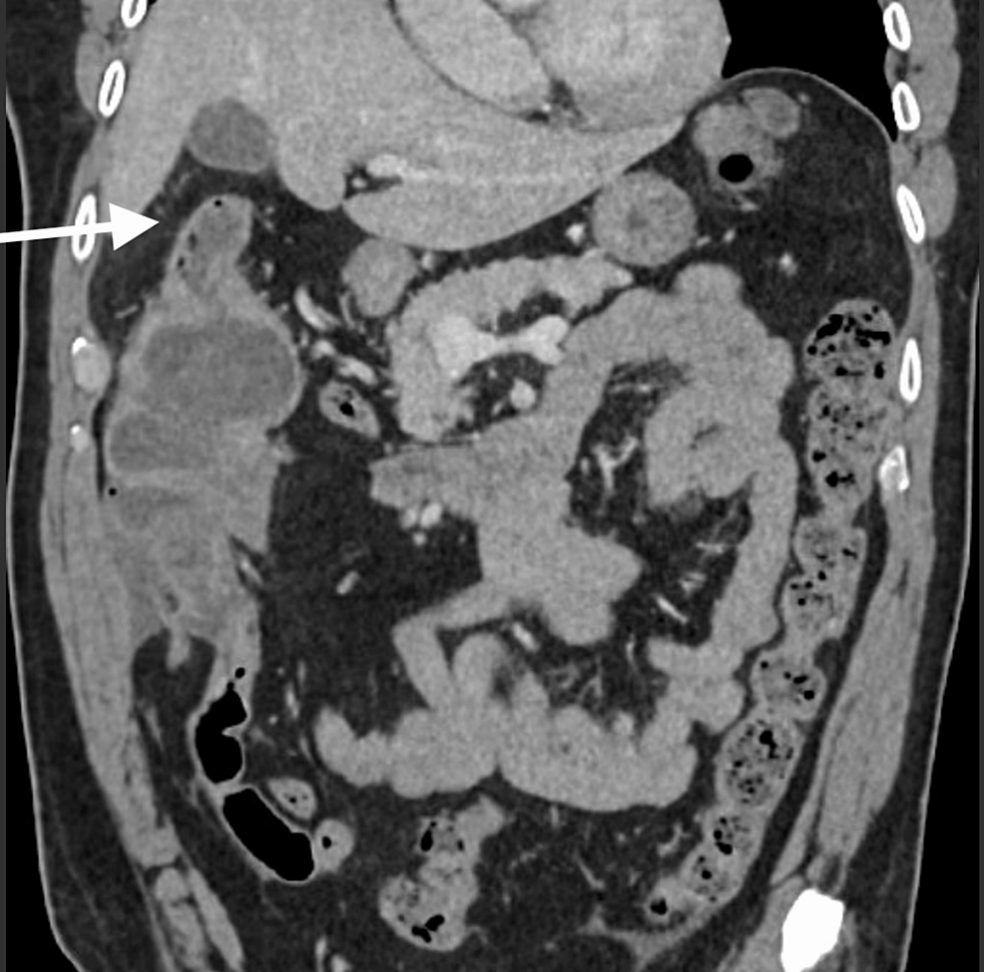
CT of the abdomen shows intraluminal hemorrhage or a mass and abrupt bowel caliber change in the vicinity of the hepatic flexure. The white arrow: an abrupt bowel caliber change in the vicinity of the hepatic flexure.
